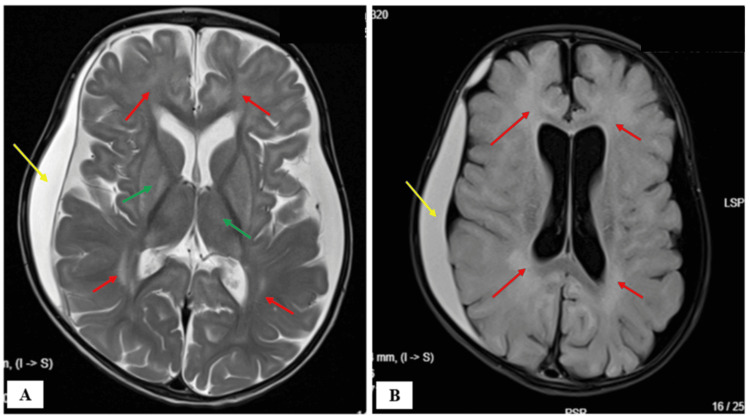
Brain MRI findings (a) Axial T2 and (b) FLAIR images show hyperintensity in the subcortical white matter of the bilateral cerebral hemisphere, more predominant in the frontal, temporal, and parietal lobes of the brain (red arrows in A and B) and bilateral basal ganglia (green arrows in A). There is a subdural hemorrhage subjacent to the right cerebral hemisphere (yellow arrows in A and B) MRI: magnetic resonance imaging; FLAIR: Fluid-attenuated inversion recovery
